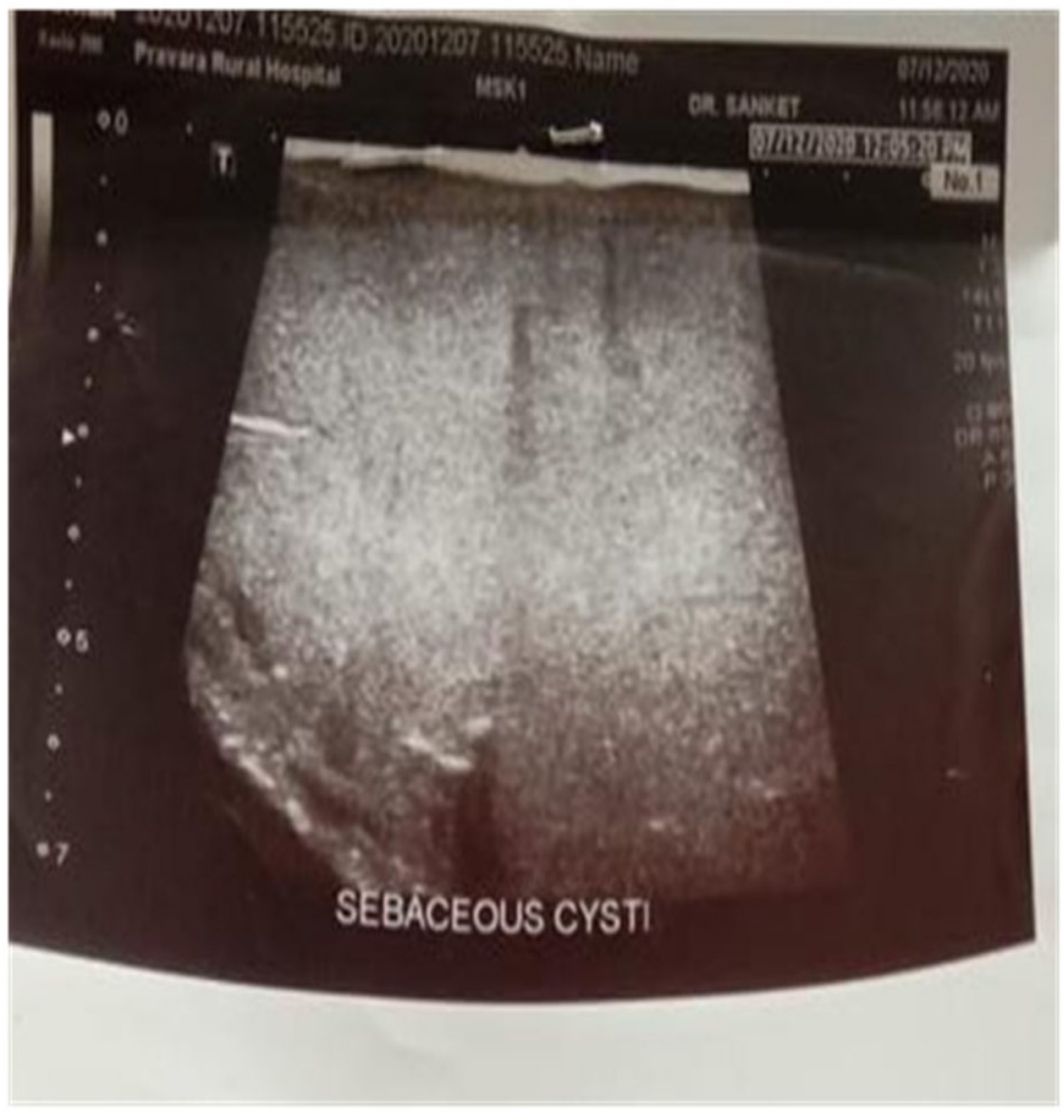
Ultrasound results showed a large, well‐defined, lobulated, and heterogeneous hypoechoic lesion with numerous small calcifications.
